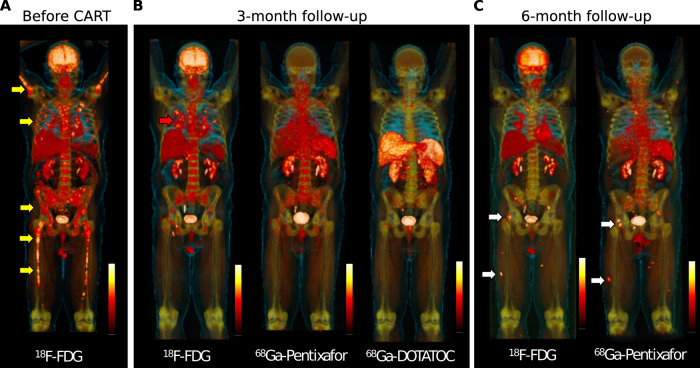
Whole body PET imaging at baseline and during follow-up using multiple PET tracers. A FDG-PET at baseline shows multiple focal lesions at the axial and appendicular skeleton (yellow arrows). B Follow-up assessment at 3-month after CAR T infusion shows full resolution of focal lesions, but residual FDG uptake located to the lung (red arrow). Matched-simultaneous CXCR4-targeted Pentixafor and DOTATOC PET did not show focal uptake. C Matched-simultaneous FDG and CXCR4 PET 6 months after CAR T depicted new focal lesions at the pelvis and lower limbs in line with relapse (white arrows).
